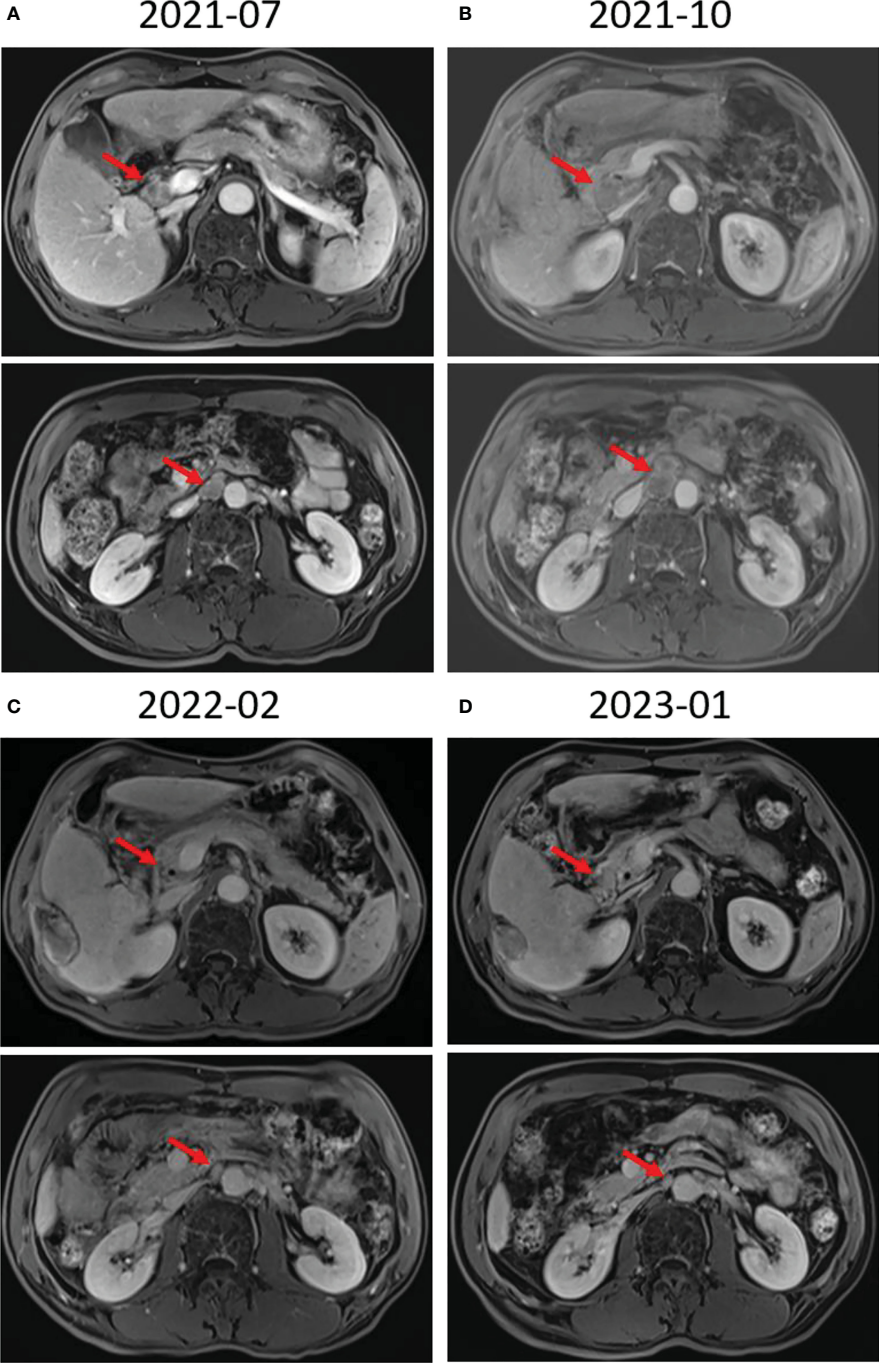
Radiological response evaluation of abdominal lymph nodes during the clinical course. (A) Contrast-enhanced magnetic resonance imaging (MRI) revealed multiple enlarged abdominal lymph nodes (red arrow). (B) Contrast-enhanced MRI showed that there were still multiple enlarged abdominal lymph nodes (red arrow) 3 months after surgical resection of lymph nodes in the hilar area. (C) Contrast-enhanced MRI showed that the size of the abdominal lymph nodes was reduced after RFA and four cycles of tislelizumab treatment (red arrow). (D) A significant reduction in the size of the abdominal lymph nodes (≤10 mm) was seen 15 months after tislelizumab treatment initiation (red arrow).
